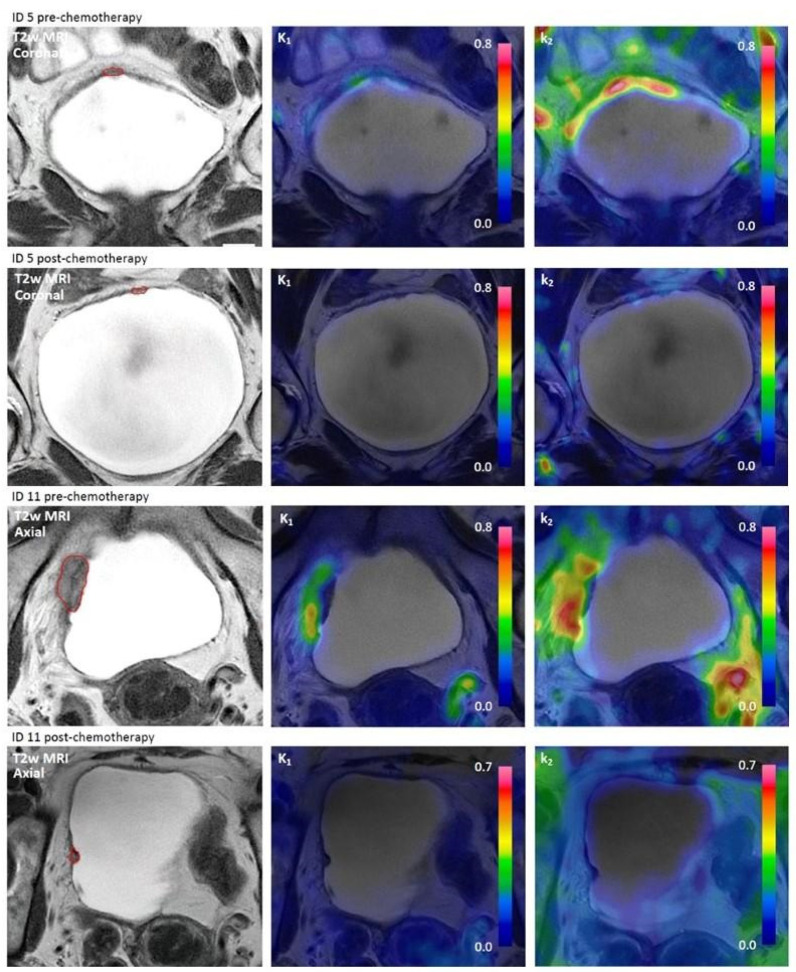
T2-weighted (T2w) MRI and [^15^O]H_2_O PET parametric images (K_1_ and k_2_) pre- and post-chemotherapy of three study patients. ID 4 had wall thickening on T2w MRI but decreased tumor blood flow (TBF) post-chemotherapy and had ypT1b + Tis. ID 5 had no visible tumor and decreased TBF post-chemotherapy and had ypT0. ID 11 had suspect volume of interest but decreased TBF post-chemotherapy, and patient had ypT0.
